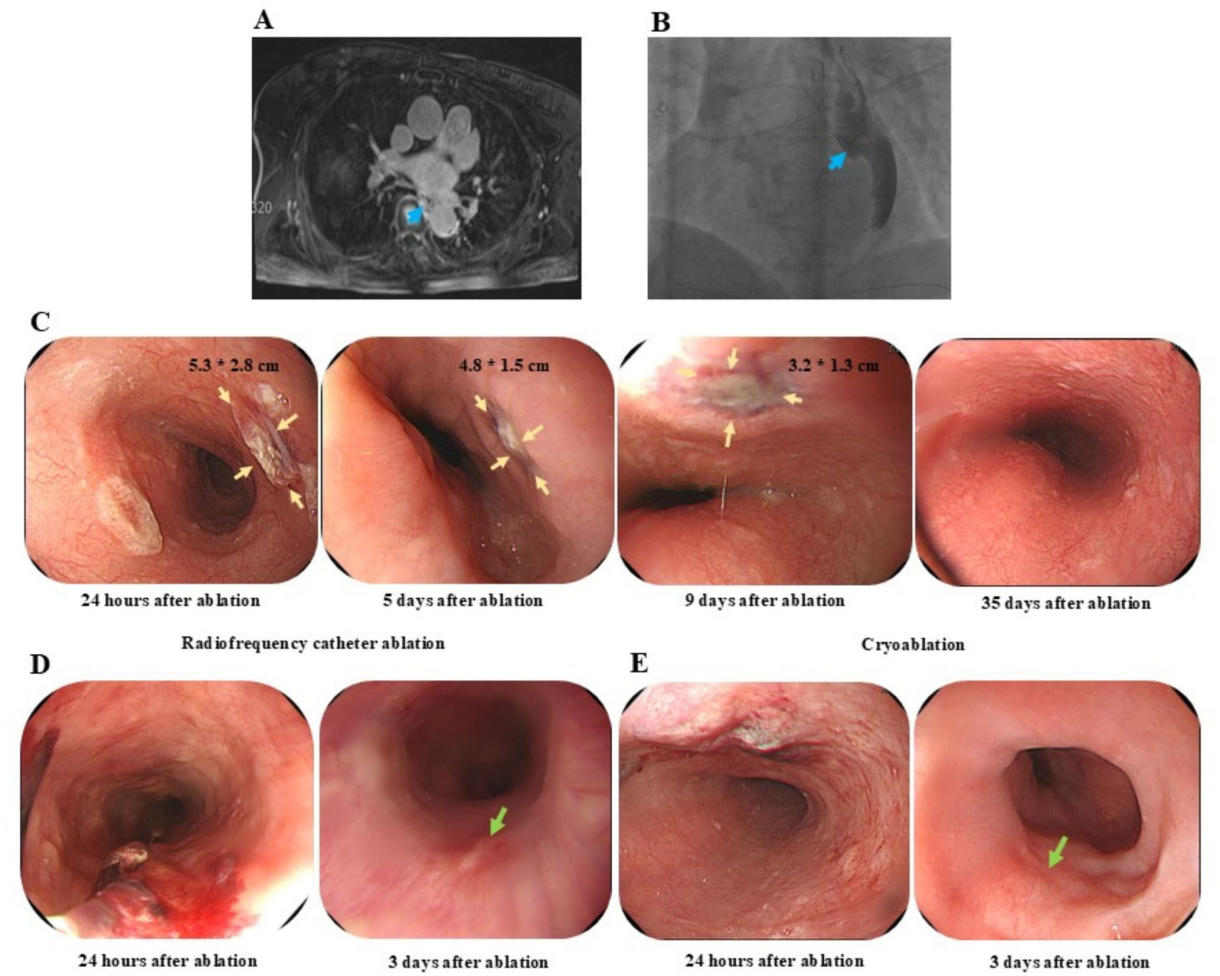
The healing process of esophageal injury. Healing of EI in patients treated with CA. (A) MRI showing compression of the esophagus by the LIPV. (B) Esophageal position confirmed by barium esophagography; most of the esophagus lies on the left side (blue arrow). (C) Endoscopic images post‐RFCA: On day 1, two superficial ulcerative lesions (largest: 5.3 × 2.8 cm) with surrounding congestion and edema were observed; high‐dose PPI therapy was initiated. By day 5, edema decreased. By day 9, lesions were smaller, and by day 35, complete healing was confirmed (yellow arrow: EI). (D, E) Comparison of lesions after RFCA and CBA. (D) RFCA‐induced superficial ulcer with hematoma. (E) CBA‐induced ulcerative lesion. EGD after 3 days of PPI therapy showed mucosal improvement (green arrow). No AEF developed. AEF, atrial‐esophageal fistula; CA, catheter ablation; CBA, cryoballoon ablation; EGD, esophagogastroduodenoscopy; EI, esophageal injury; LIPV, left inferior pulmonary vein; MRI, magnetic resonance imaging; PPI, proton pump inhibitor; RFCA, radiofrequency catheter ablation.
